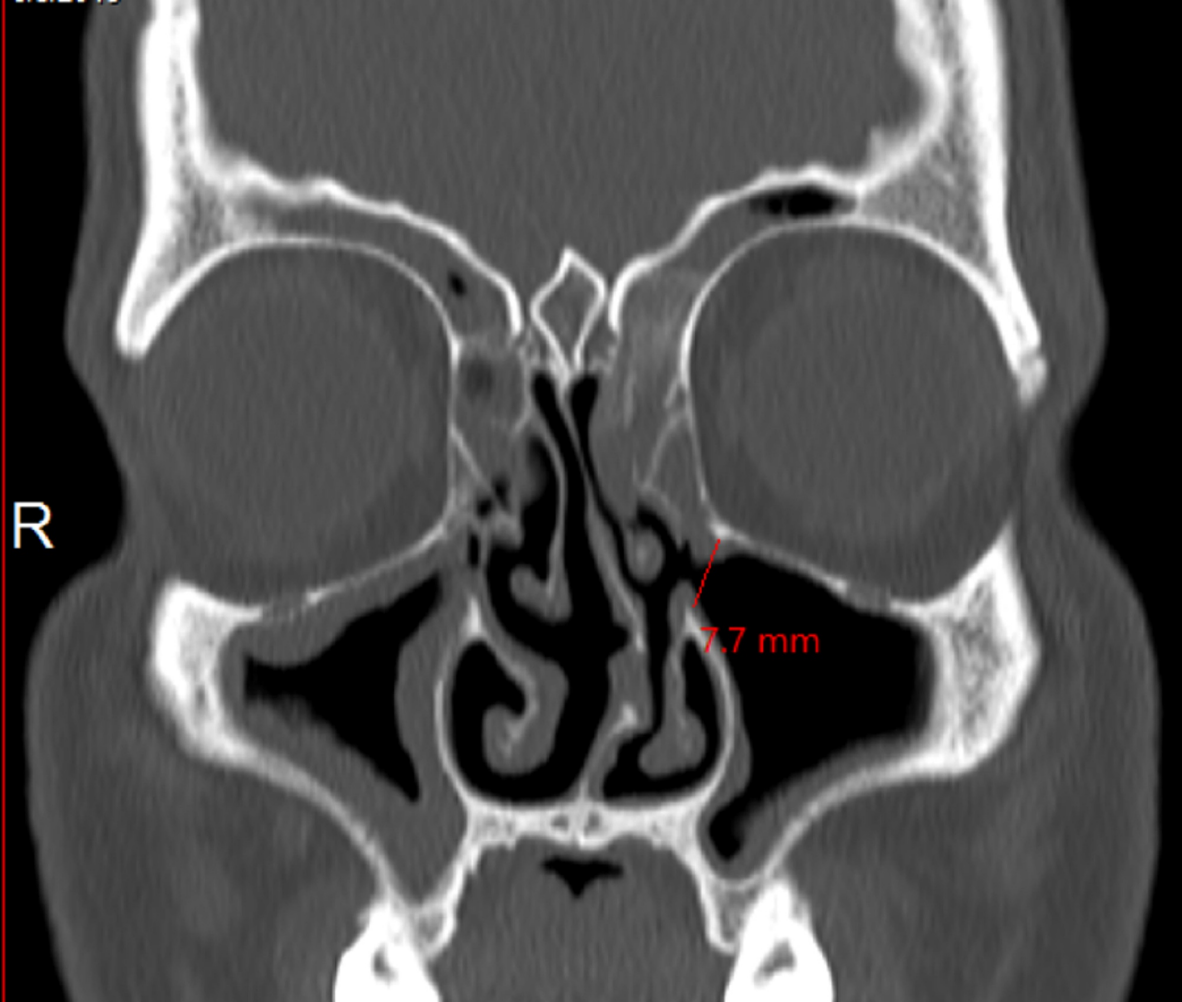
CT scan of paranasal sinus shows left AMO measuring 7.7 mm. CT: computed tomography, AMO: accessory maxillary ostium.
